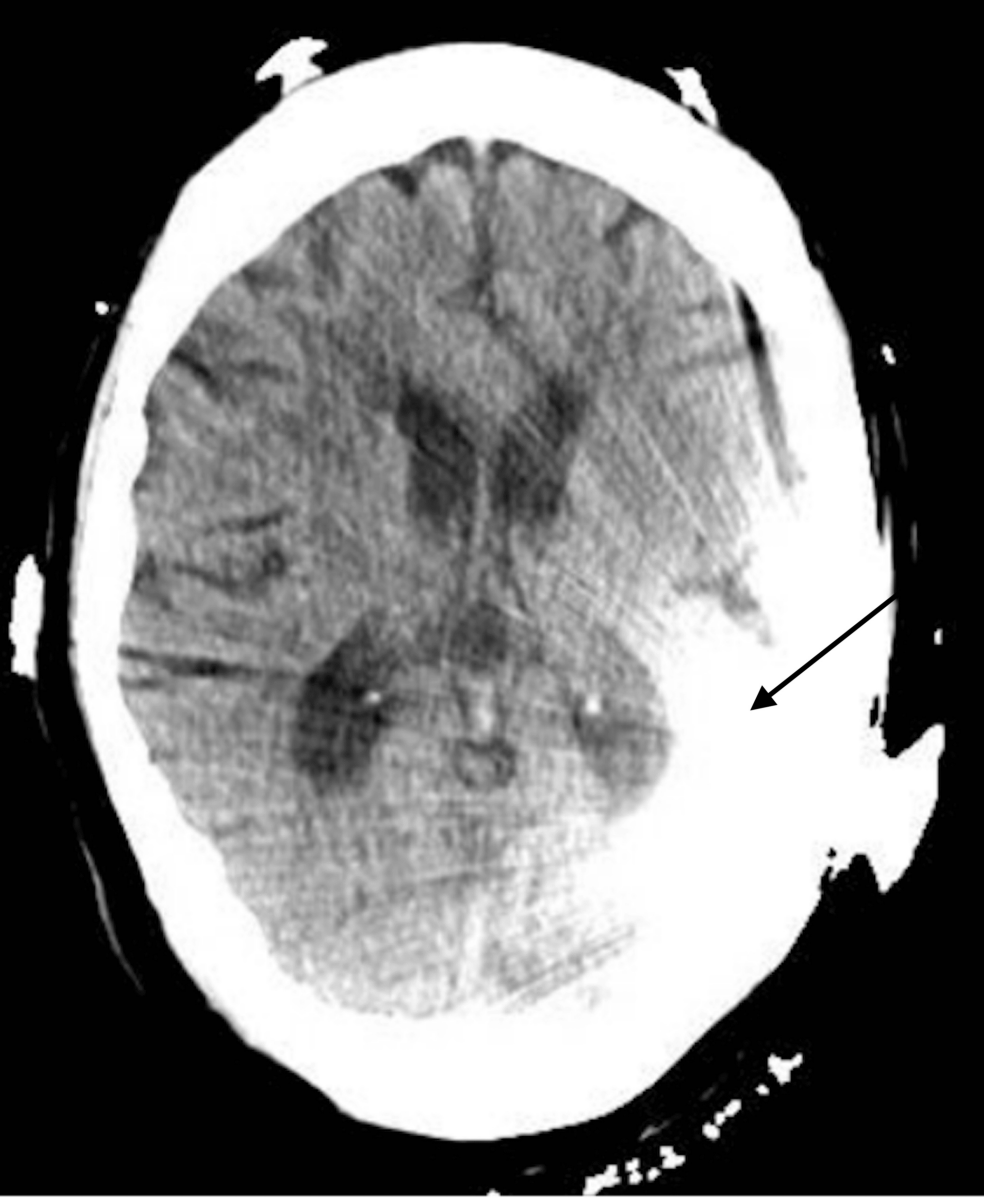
CT Head showing an artifact with cochlear implant
